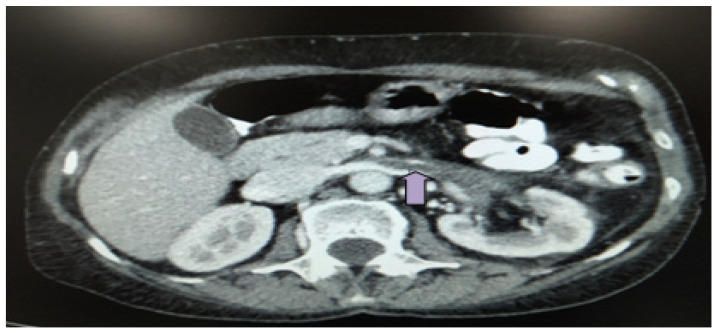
Abdominal CT scan/angiography upon presentation documenting acute thrombosis of the left renal (arrow) and ovarian vein with subsequent development of collateral capsular venous network (axial level).
